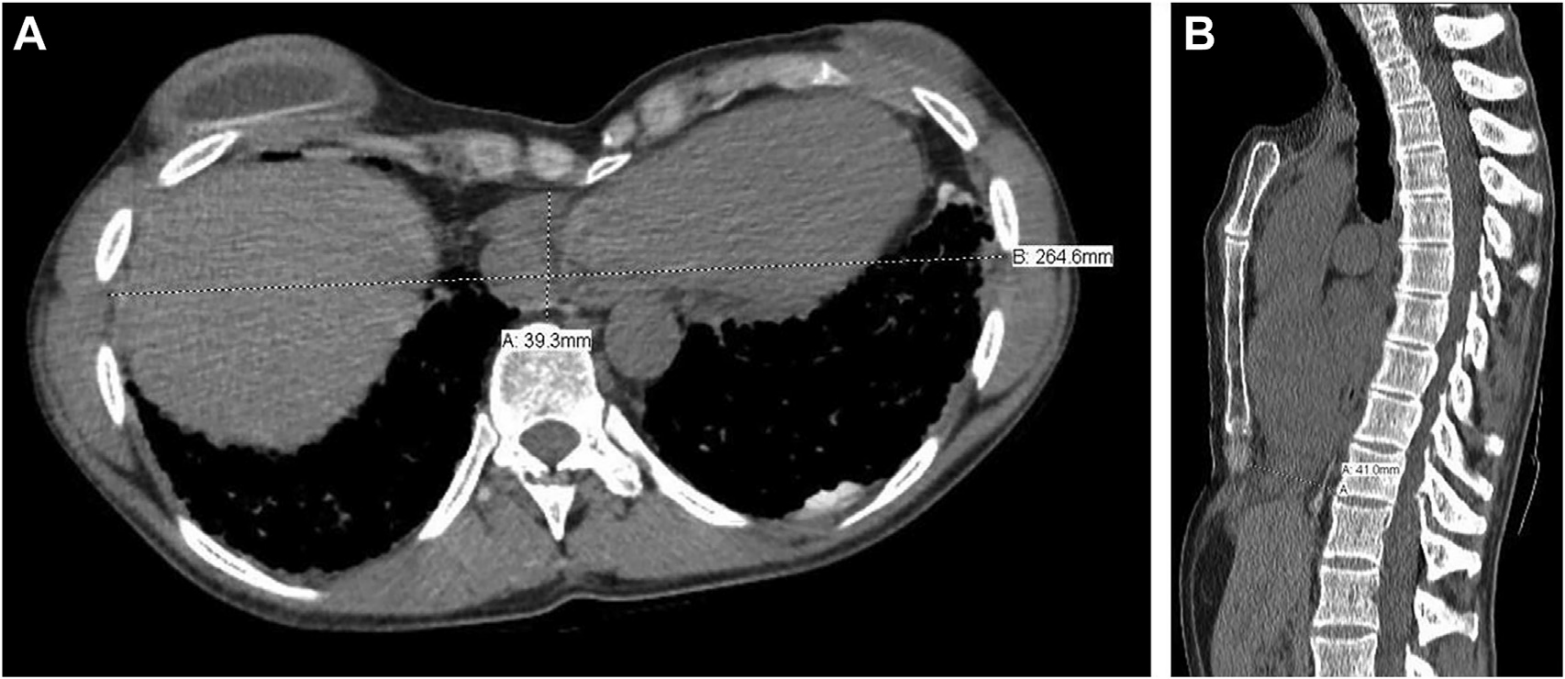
Preoperative computed tomographic scan demonstrates severe pectus excavatum with a Haller Index of 6.7. (A) Axial view. (B) Sagittal view.
